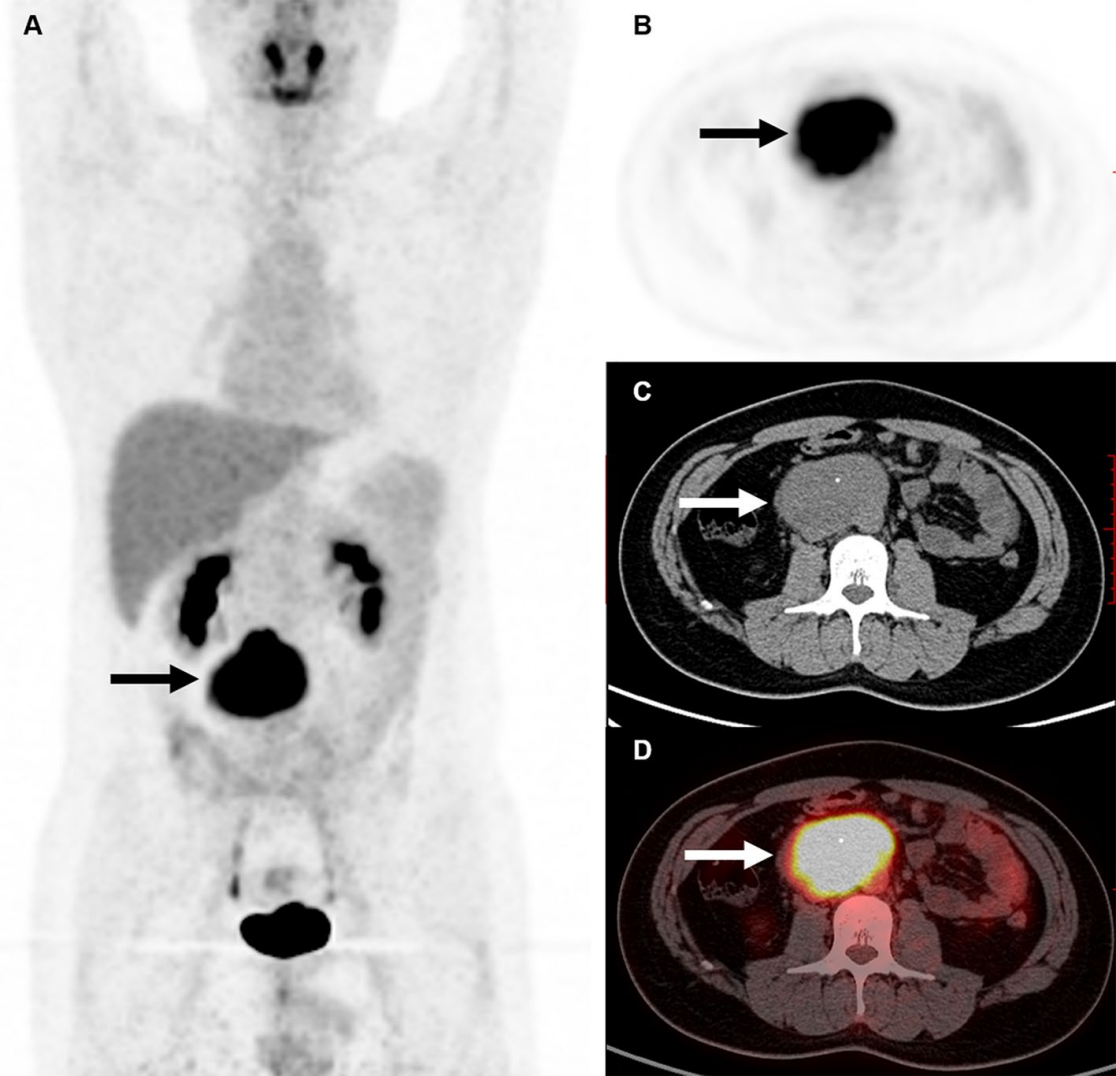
Representative case of a 32-year-old man with a retroperitoneal paraganglioma (patient 6). The patient underwent ^18^F-FDG PET/CT for characterizing the nature of the retroperitoneal solid mass incidentally detected by abdominal CT in a routine physical examination. The mass showed intense FDG activity with an SUVmax of 15.2 and a size of 8.1 cm (arrows).
